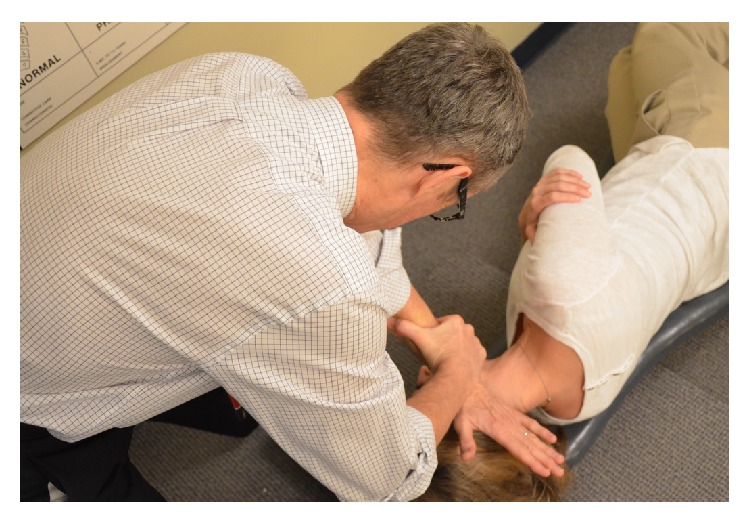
Making a NUCCA correction. The NUCCA practitioner delivers a triceps pull adjustment. The practitioner's body and hands are aligned to deliver an atlas correction along an optimal force vector using information obtained from radiographs.
